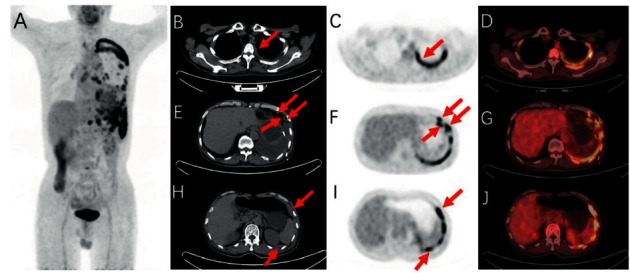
A 71-year-old man presented with cough and was diagnosed with PMLAC by thoracoscopic biopsy, whose LAC lesion was undiscovered by CT or 18F-FDG PET/CT. (a) Mixed 18F-FDG uptake of the left pleura. (c, d) Diffused 18F-FDG uptake of the left pleura. (b) Lamellar thickening of the left pleura. (f, g, i, j) Focal 18F-FDG uptake of the left pleura. (e, h) Multiple nodules on the left pleura. All are indicated by the red arrow.
